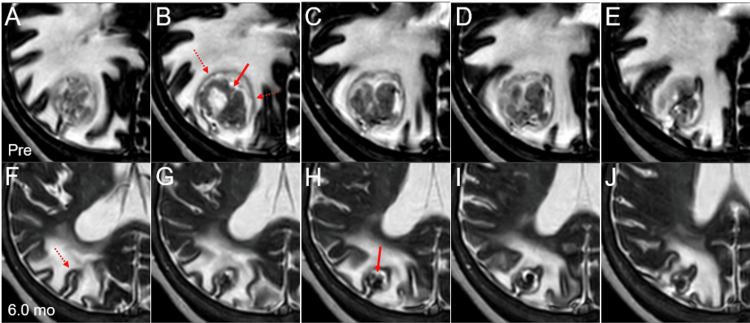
T2-weighted images before and six months after stereotactic radiosurgery. The images show axial T2-WIs (A-J); before SRS (A-E); and at 6.0 months after SRS (F-J). (A-J) All images were co-registered and are shown in the same magnification and coordinates. (A-E) An irregularly-shaped mass (arrow in B) is surrounded by a slightly high-intensity rim (dashed arrows in B), with a high-intensity structure, possibly trapped cerebrospinal fluid, intervening these structures. (F-J) At 6.0 months after SRS, the regressed low-intensity irregular mass with continuity to part of the cortexes is mainly located between the cortexes in the intraparietal sulcus (dashed arrow in F). WI: weighted image; SRS: stereotactic radiosurgery
